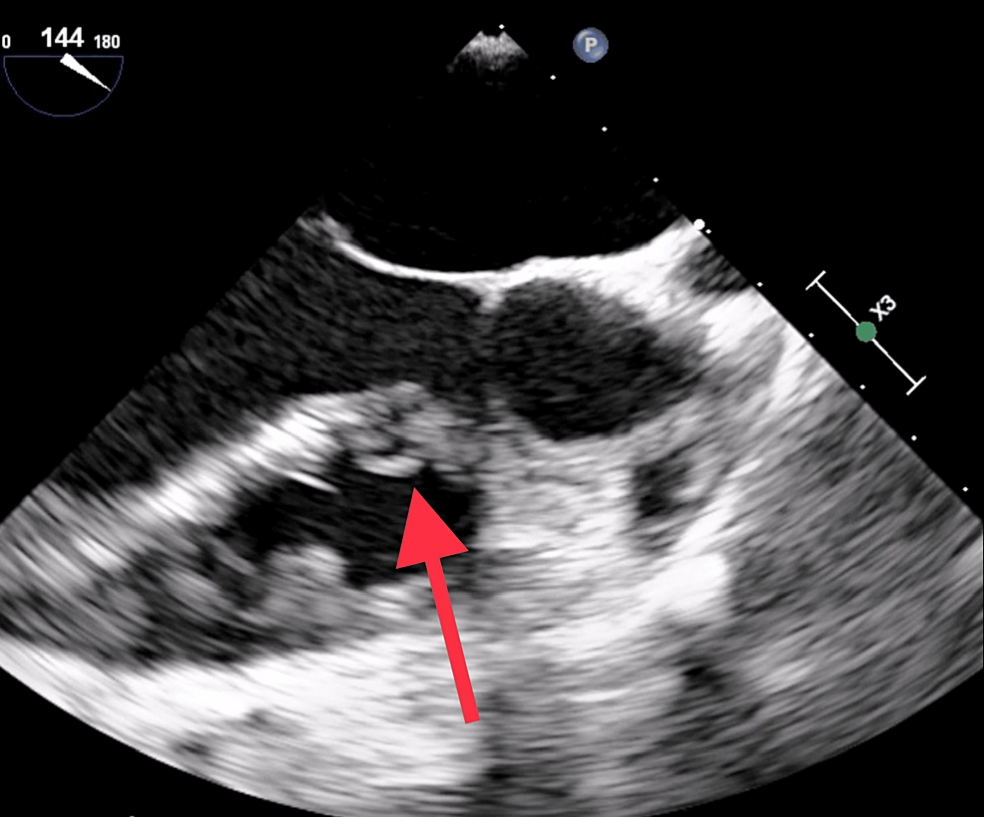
Transesophageal echocardiography view at angle 144 showing a mass attached to the septal wall from the right ventricular side, representing vegetation
